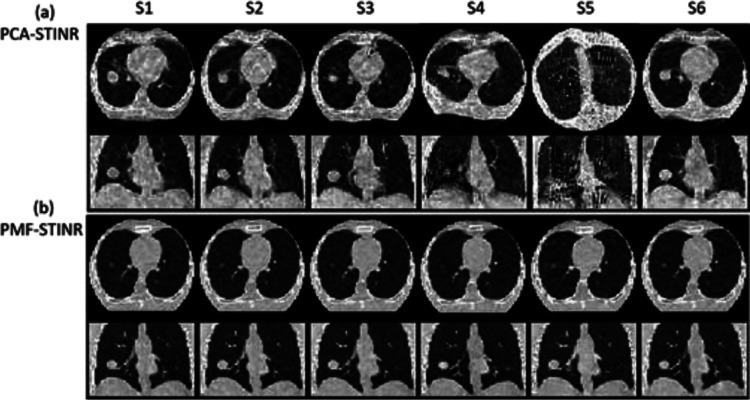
Comparison of reference-frame CBCTs reconstructed by (a) PCA-STINR and (b) PMF-STINR for the six motion scenarios (S1–S6) in the axial and coronal views.
